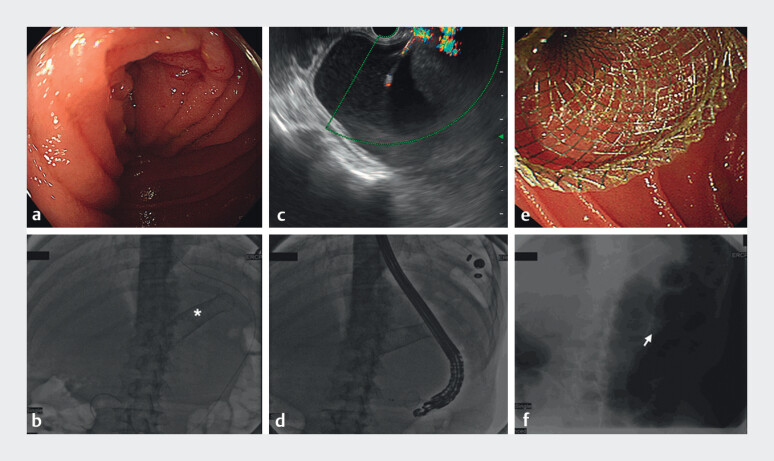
Endoscopic ultrasound-guided enteroenterostomy with a lumen-apposing metal stent (LAMS).
